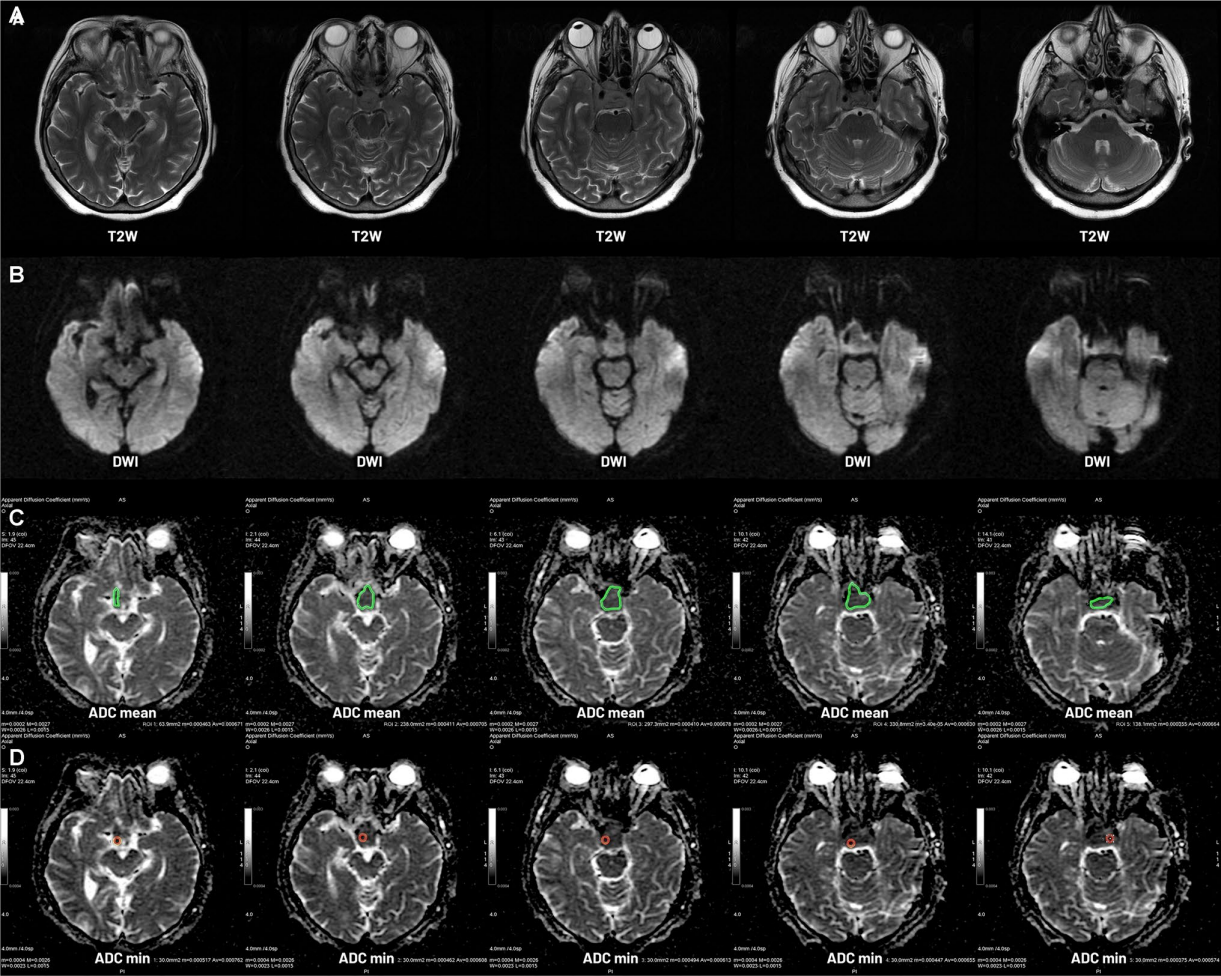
Axial T2-weighted images present a sellar and suprasellar meningioma (A), which is also visible on DWI images (B). The mean ADC measurements are obtained by outlining the whole lesion with a region of interest (ROI) on all axial slices of the ADC map that constrained the pathology (C). The min ADC value is the lowest value measured with an ROI of approximately 30 mm.^2^ selected from all cross-sections of the lesion (D)
